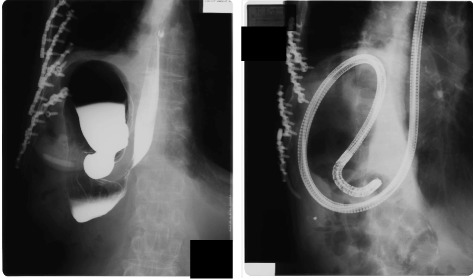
The upper gastrointestinal radiography and the upper gastrointestinal endoscope examination of case 1 The upper gastrointestinal radiography (left) shows gastric torsion with an adhesion of the gastric body in the upper side rather than the gastric fornix. The upper gastrointestinal endoscope examination (right) shows the torsional axis and position of the gastric body
